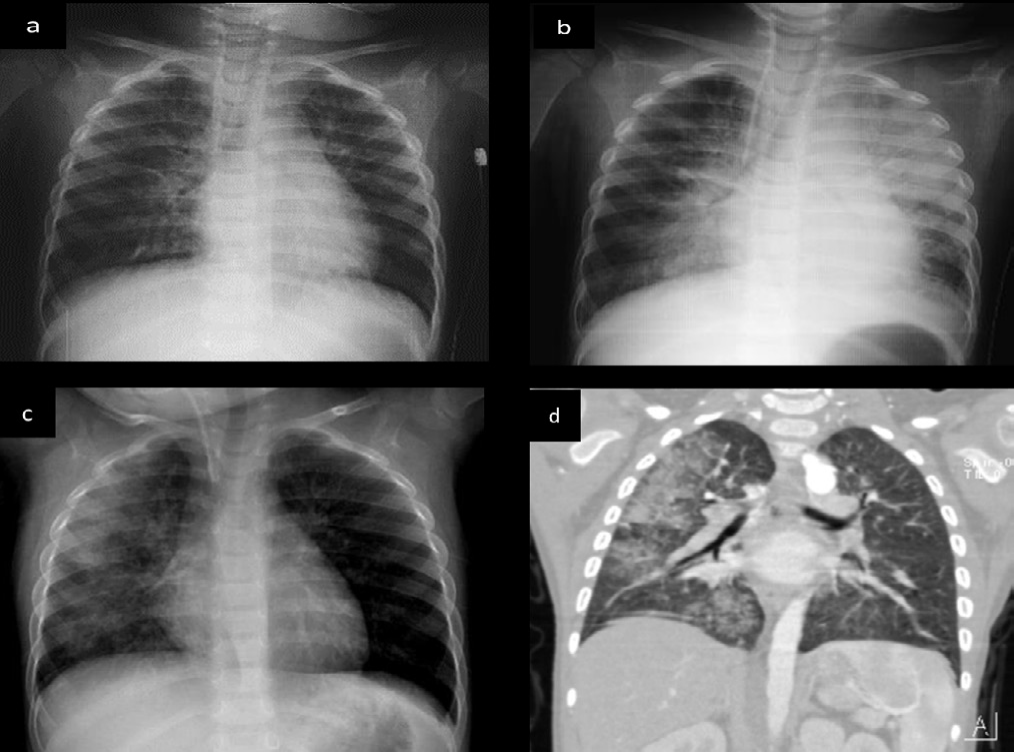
Imaging findings of a 2-year-old girl who presented with multisystem inflammatory syndrome in children associated with SARS-CoV-2, with symptoms including fever, vomiting and clinical findings of metabolic acidosis, dehydration and hypotension. (a) (initial). Frontal chest radiograph shows perihilar and paracardial peribronchial infiltration (b) (6 h later). The radiograph shows that the perihilar and paracardial peribronchial infiltration has progressed towards asymmetric, larger, consolidative opacities on the left. On radiography (c) (27 days after a and b) and tomography (d) (27 days after a and b) there was a multifocal consolidation and ground glass opacities persisting in the right lung including the cardiophrenic region.
